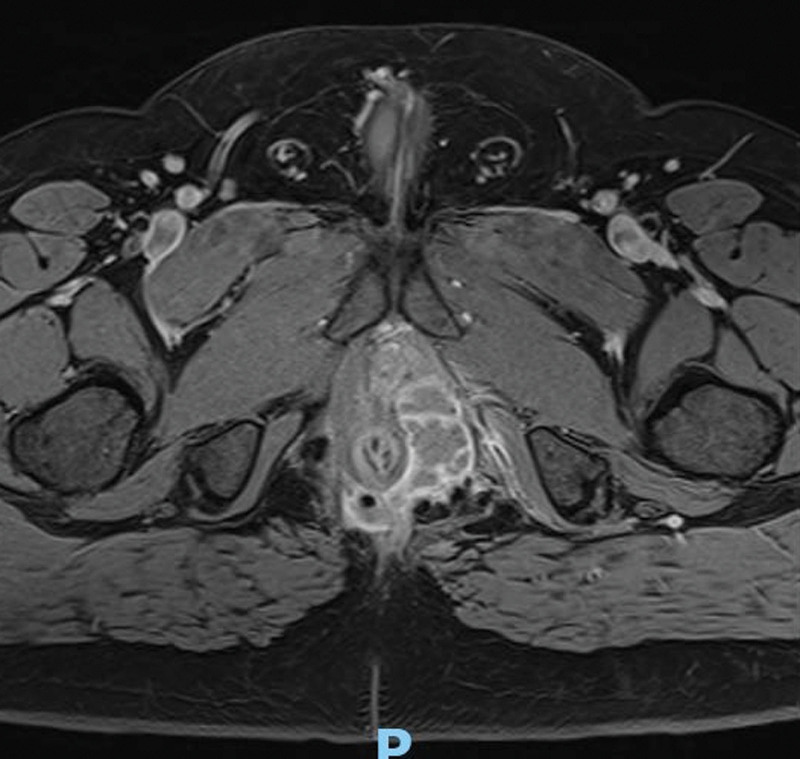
MRI showed localized large deep-seated anorectal sepsis. MRI = magnetic resonance imaging.
